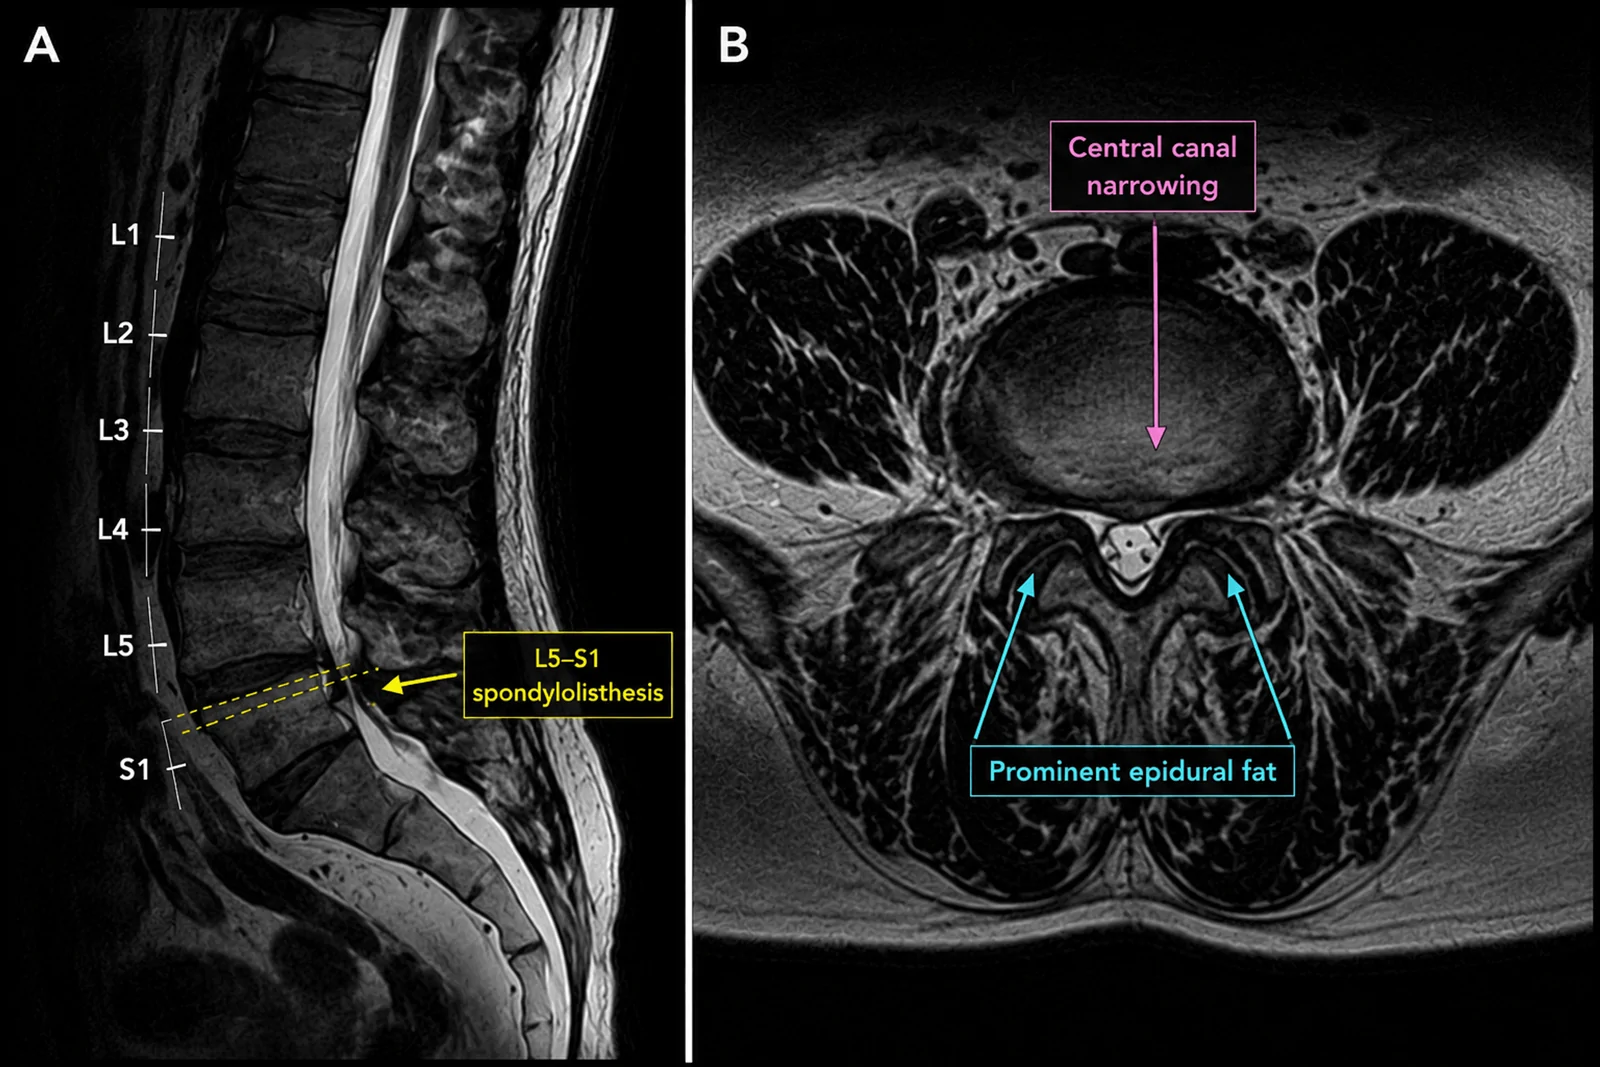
Lumbar magnetic resonance imaging findings. (A) Sagittal T2-weighted image demonstrating marked degenerative lumbar spondylosis with L5-S1 spondylolisthesis and multilevel degenerative disc degeneration. (B) Axial T2-weighted image demonstrating central canal narrowing associated with degenerative changes and prominent epidural fat. No epidural hematoma, spinal cord compression, or other acute neuraxial compressive lesion was identified on non-contrast MRI.
